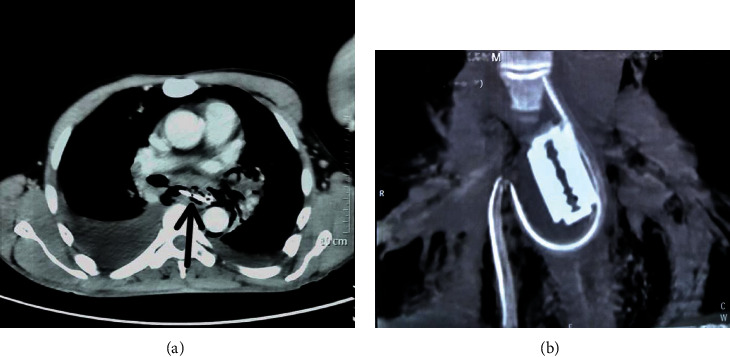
CT chest. (a) Sharp razor blade (arrow) in the mediastinum with pneumomediastinum and moderately large right pleural effusion. (b) Reconstructed image shows the whole blade; apparently, the NG tube traveled through the mediastinum.
